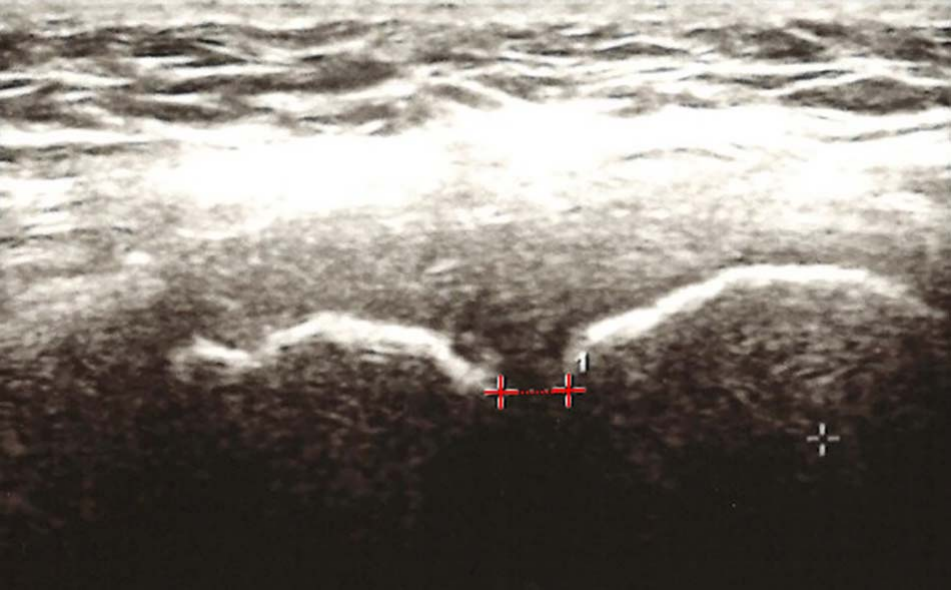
Interpubic width measurement using ultrasound imaging. The line from which the distance was measured is indicated by a dotted line.
